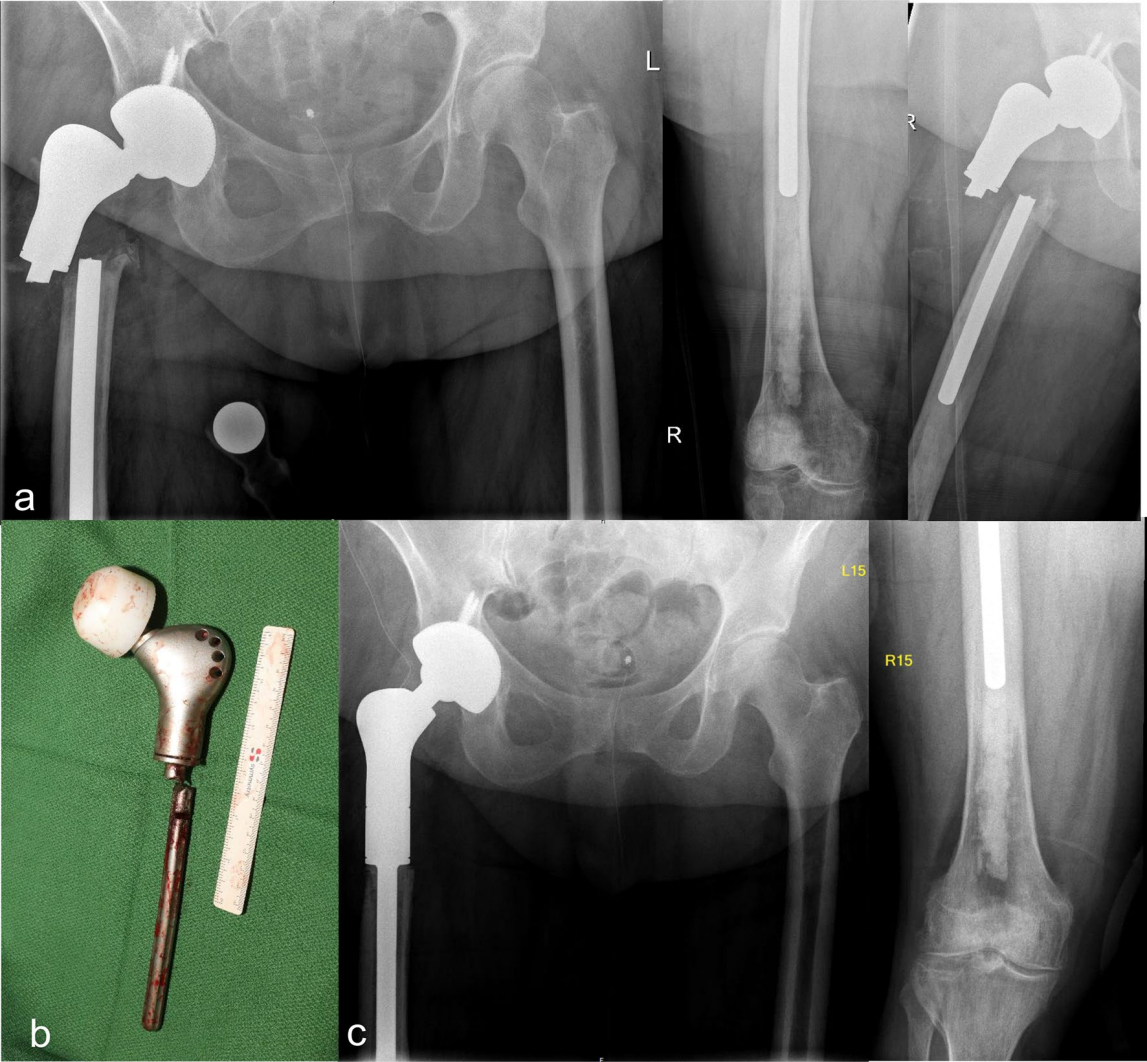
A broken implant (b), diagnosed on clinical findings and x-ray imaging (a), that was cared for in a single stage replacement with explantation and reimplantation with cement in cement (c).
